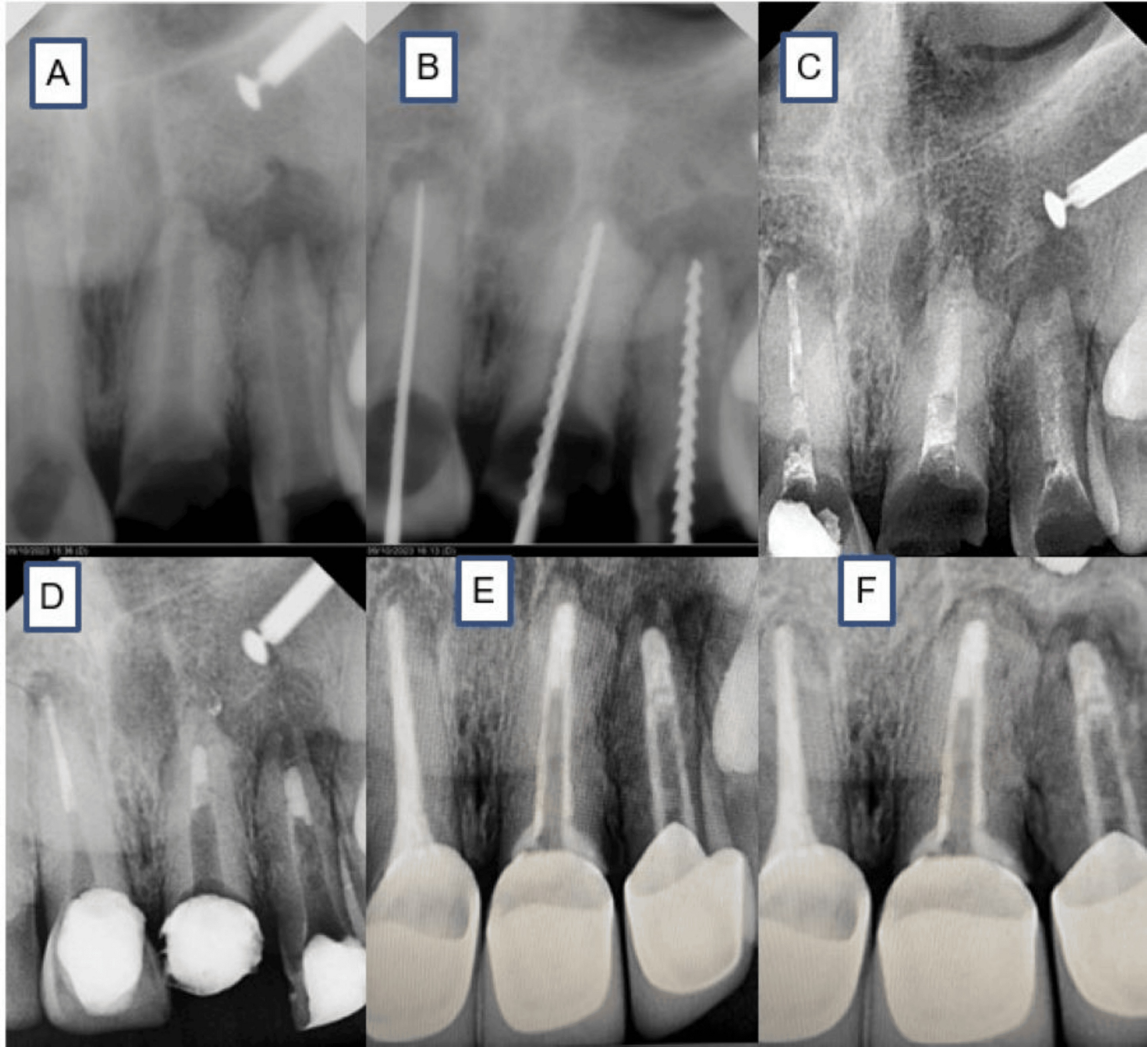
Radiographic representation (A) Pre-op. (B) Working length determination. (C) Ca(OH)2 dressing placed. (D) A-PRF+Biodentin placed. (E) One-month post-op. (F) Nine months follow-up Ca(OH)2: calcium hydroxide; A-PRF: advanced platelet-rich fibrin
